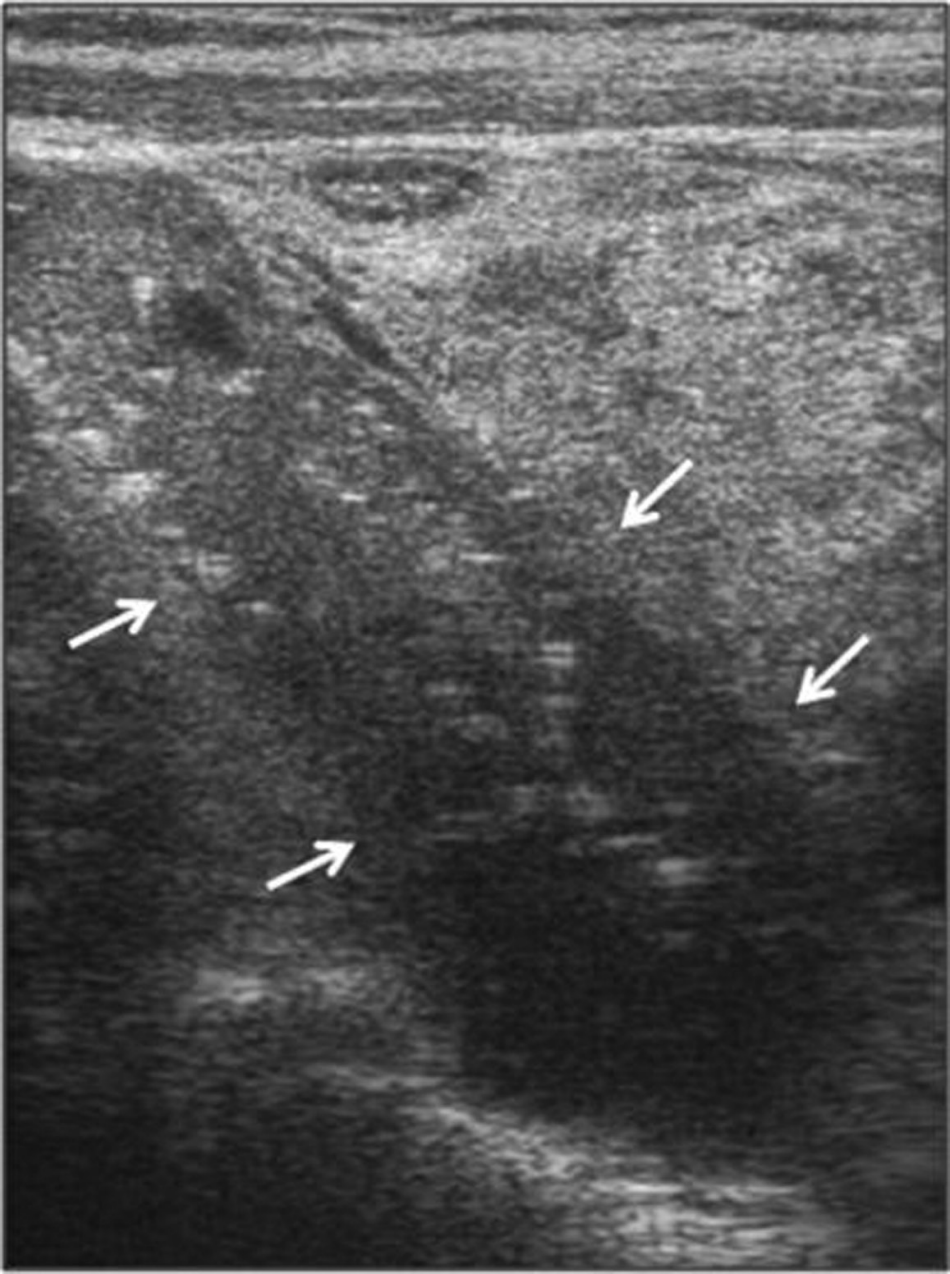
Ultrasound image of a parathyroid carcinoma. The tumor (arrows), located at the upper pole of the thyroid, shows a heterogeneous pattern, irregular shape and halo sign (longitudinal view) (from Cetani *et al*. JEI 2016, with permission (18)).
